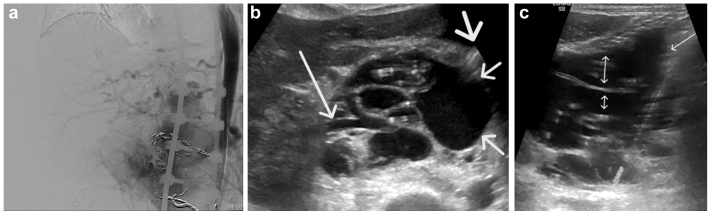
Patient 7 had a complex retroperitoneal arteriovenous malformation (AVM). (A) Abdominal angiogram demonstrating extensive retroperitoneal arteriovenous shunting centered around the right renal hilum. (B) Transverse ultrasound image demonstrating the complex right retroperitoneal arteriovenous shunt posterolateral to the inferior vena cava (IVC) (*thin arrows*). Note the needle approaching from the left (*thick arrow*), and common hepatic artery traversing the malformation (*long arrow*). (C) Transverse ultrasound image demonstrating the transcaval course of the needle. Note tenting of the anterior wall of the IVC and large veins directly draining into the IVC (*double arrow heads*).
